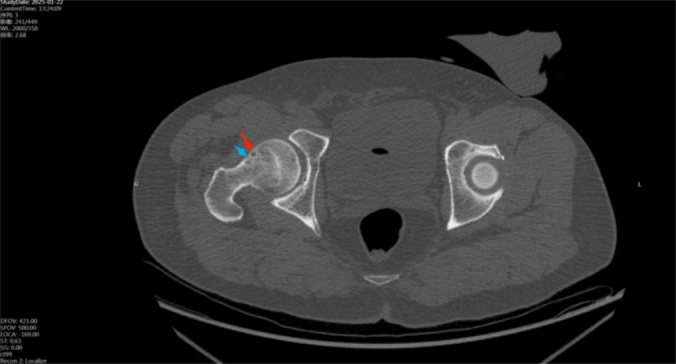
Preoperative hip CT revealed no definite fracture line in the femoral neck. A small cystic lucency (∼3 mm) was noted in the anterior midportion of the femoral neck, suggestive of a bone cyst or synovial hernia pit (arrow).
